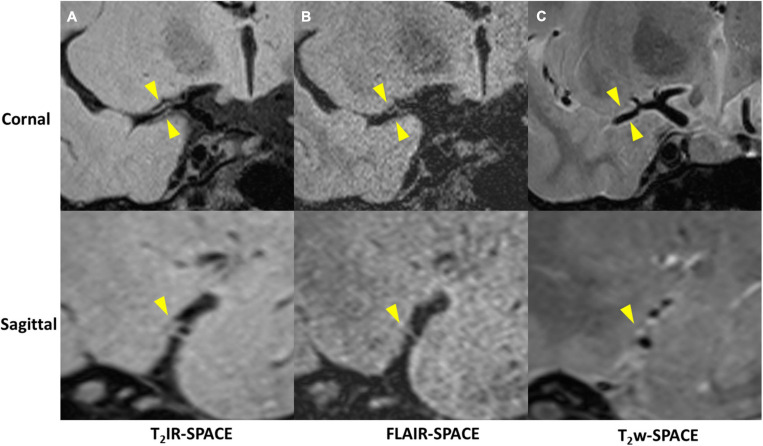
A 33-year-old female patient diagnosed with vasculitis. (A) T_2_IR-SPACE, (B) FLAIR-SPACE, and (C) T_2_w-SPACE images. Top: coronal view of the right MCA; Bottom: sagittal view of the thickened vessel wall pointed by the arrowheads. Among the three T_2_w sequences, only T_2_IR-SPACE depicted concentric wall thickening on the M1 segment of the right MCA; the vessel wall was not clear on FLAIR-SPACE, and most parts of the vessel wall were missing; the vessel wall on T_2_-weighted SPACE imaging appeared normal.
